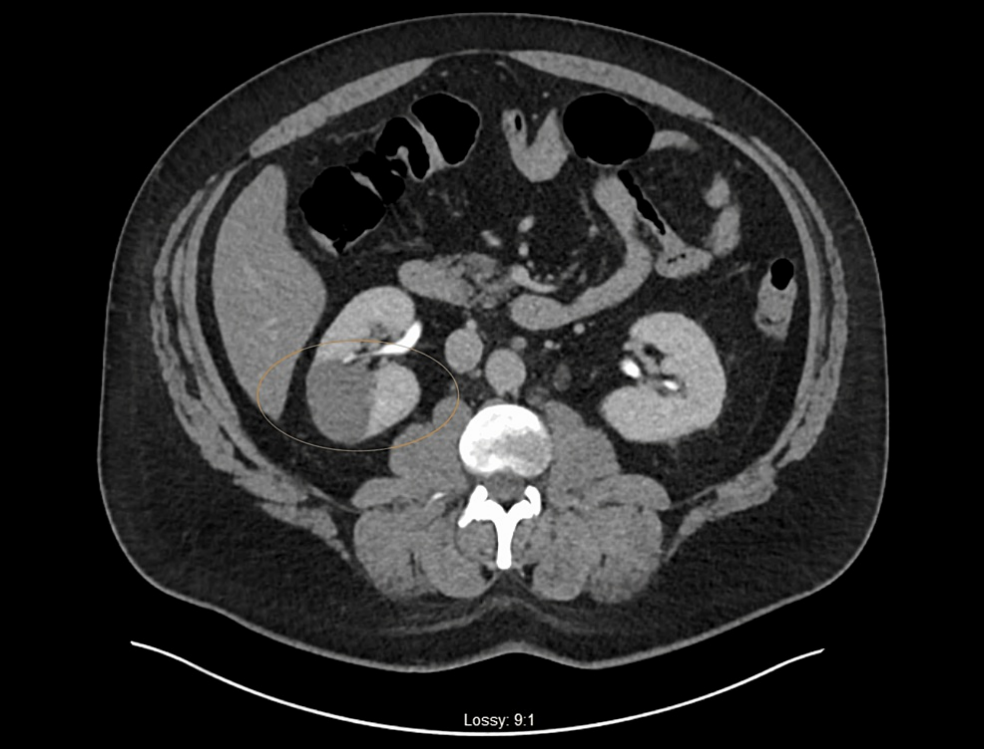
CT urogram showing right renal infarction
